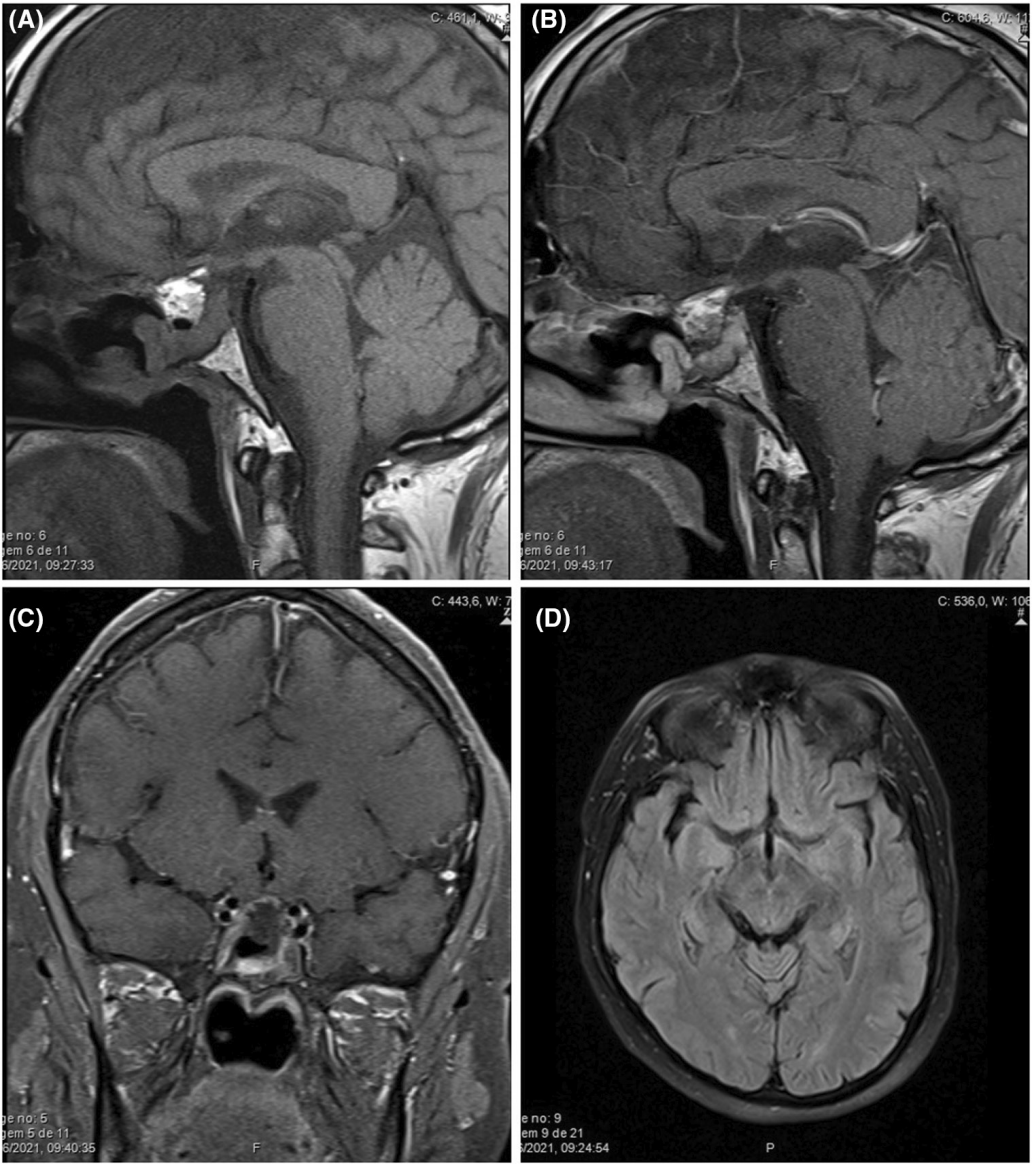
Post‐operative MR sagittal T1 (A) and T1 post‐gadolinium (B), coronal T1 fat suppressed post‐gadolinium (C), axial T2 FLAIR (D) scans: complete removal of the sellar and suprasellar lesion with fat filling of the surgical space (T1 hyperintense, hypointense in the T1 fat suppressed sequence), and complete resolution of the optic pathway edema (T2 FLAIR).
